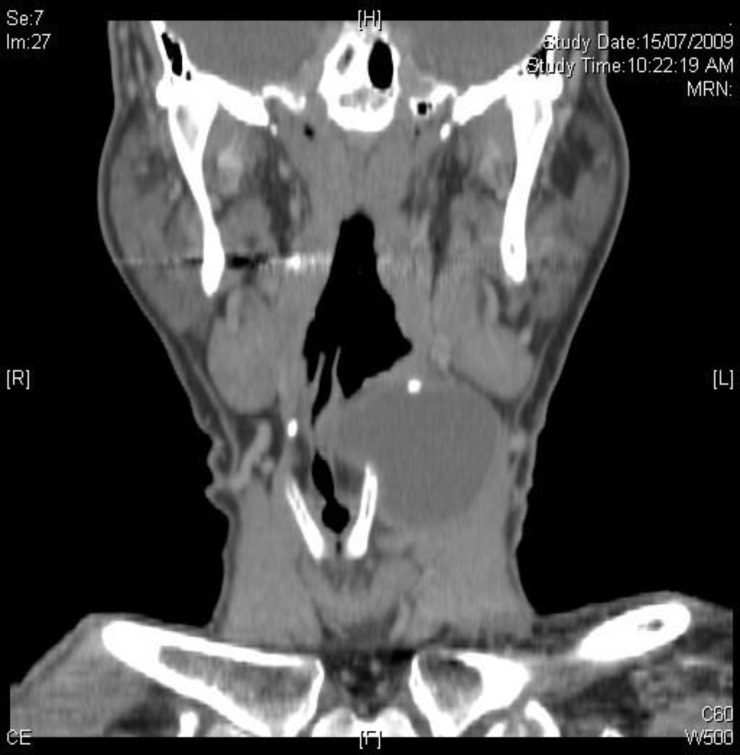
Coronal CT scan, demonstrating cystic lesion over the left side of the neck.
